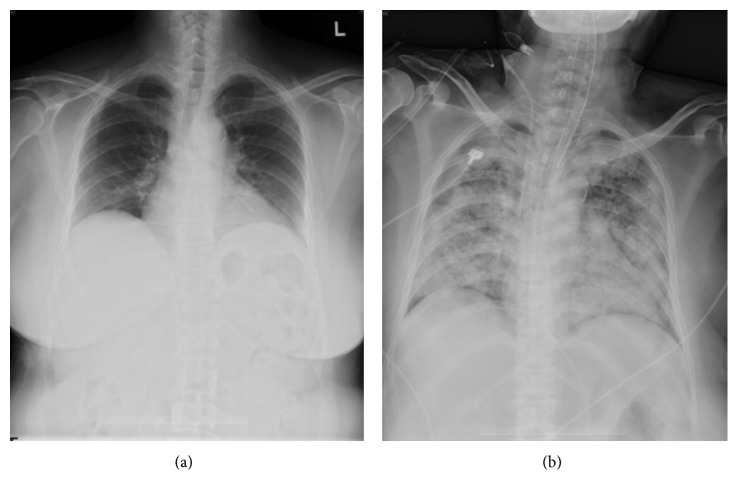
Chest radiograph revealed ARDS lung. (a) preoperative; (b) postoperative.
